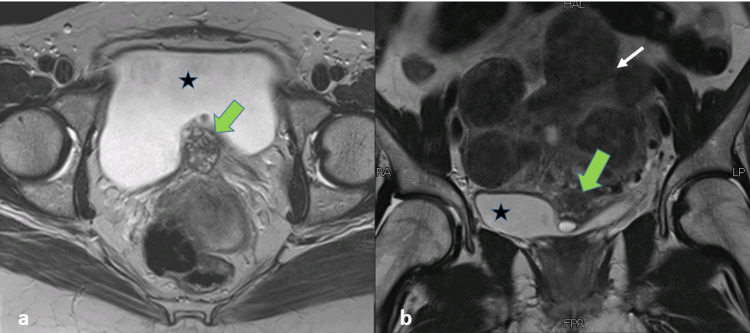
MRI pelvis - image 1 (a) Axial T2-weighted image and (b) coronal T2-weighted image demonstrate heterogeneous ill-defined predominantly T2 hypointense lesion (green arrow) along the base of the urinary bladder (star sign) with contour abnormality. Fibroid uterus are shown on the coronal view (white arrow) MRI: magnetic resonance imaging
